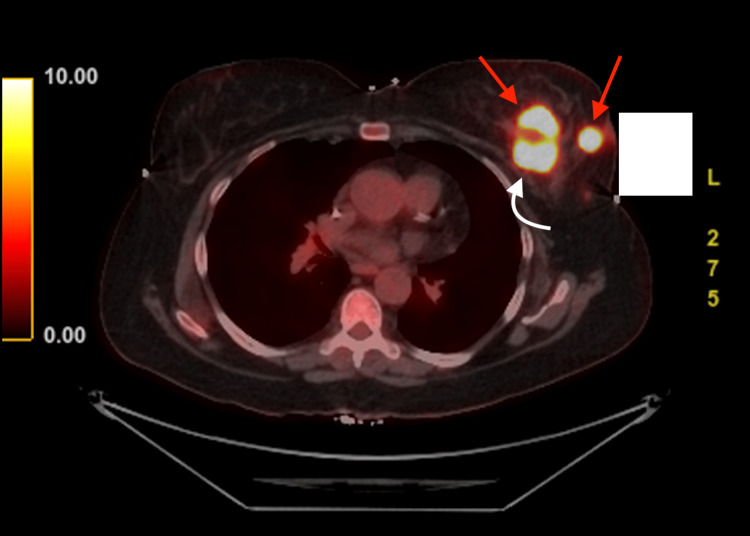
Axial fused PET-CT This image depicts an axial fused PET-CT demonstrating multiple hypermetabolic lesions (red arrows) within the left breast and extending to the chest wall (white arrow). PET-CT: positron emission tomography-computed tomography
